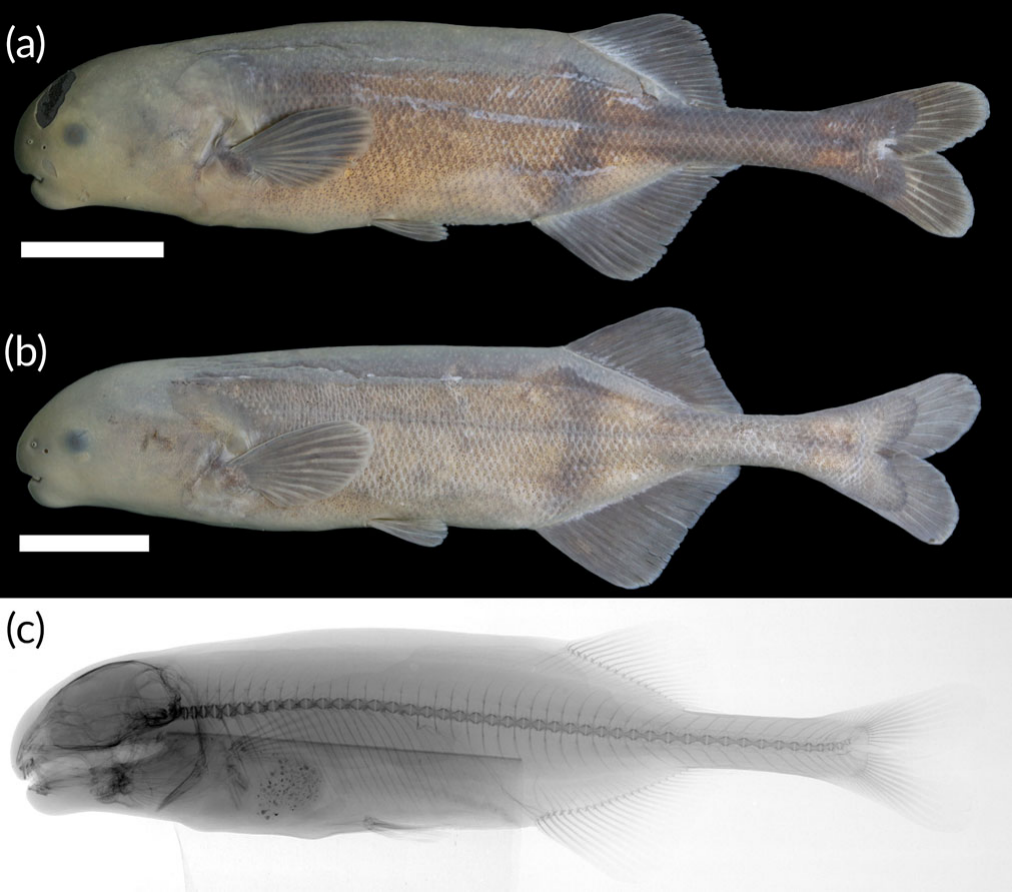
(a) Holotype of *Heteromormyrus chilembwei* sp. nov. (SAIAB 119004), 65.0 mm standard length (SL), from the Ruo River, lower Zambezi River system, Malawi, southern Africa; (b) paratype of *H. chilembwei* sp. nov. (SAIAB 119004), 69.0 mm SL, from the Ruo River, lower Zambezi River system, Malawi, southern Africa; (c) X‐ray radiograph of holotype. Scale bar =1 cm.
